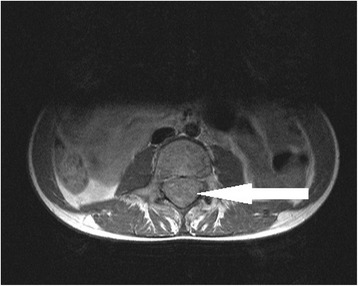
MRI of the spine. Axial T1-weighted post-contrast injection image: the mass occupies the entire cross-section of the spinal canal
